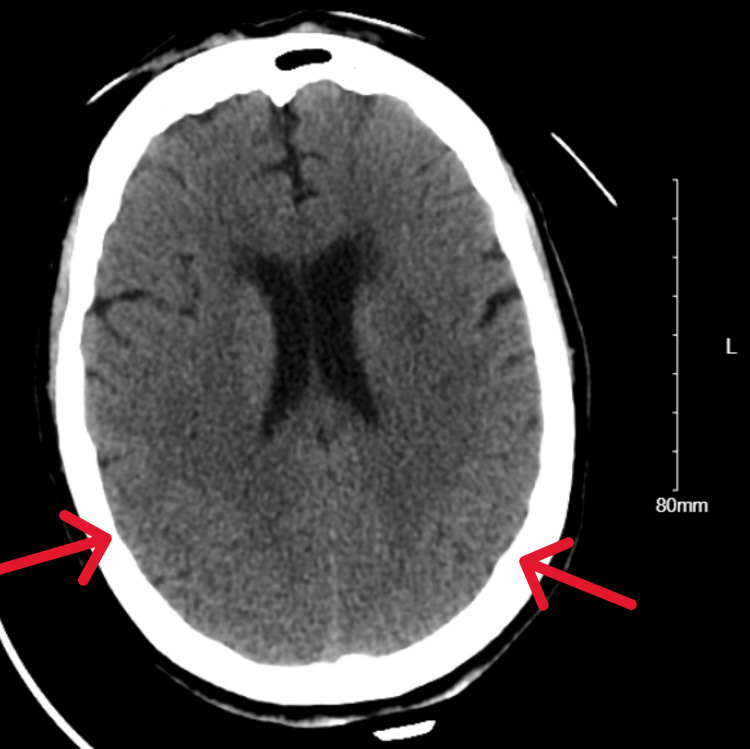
CT of the head w/o contrast showing bilateral sulci effacement CT: computed tomography
